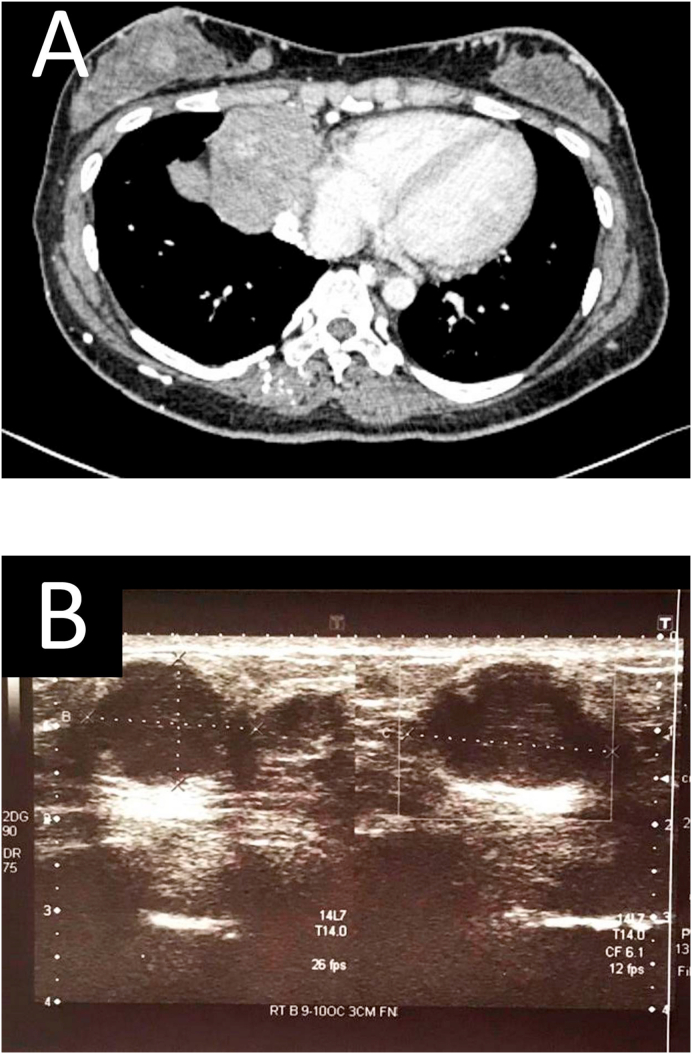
Chest CT revealing an enhancing right breast nodule (A). Ultrasound of breast (B) showing irregular hypoechoic breast lesions.
